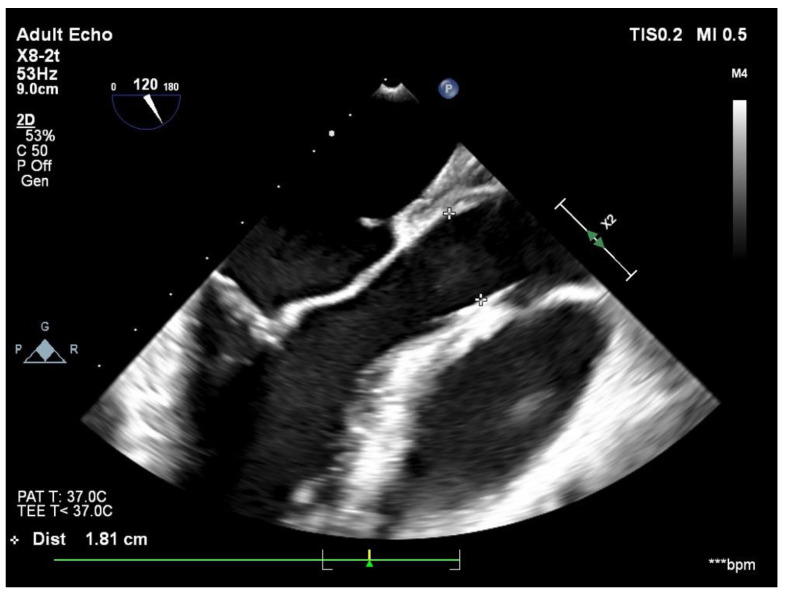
Transesophageal echocardiographic preoperative representation.
